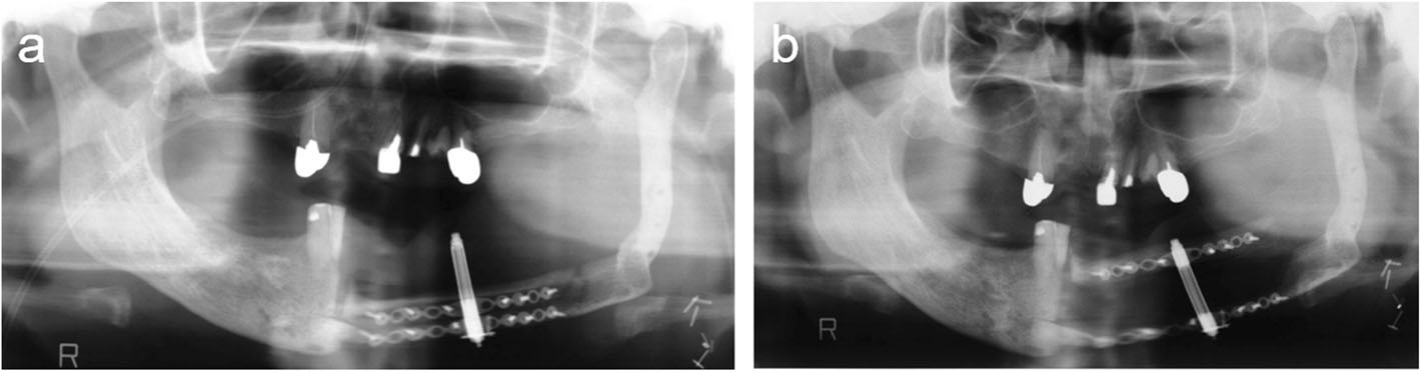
Orthopantomogram a 1 day postoperatively and b at the end of distraction osteogenesis
